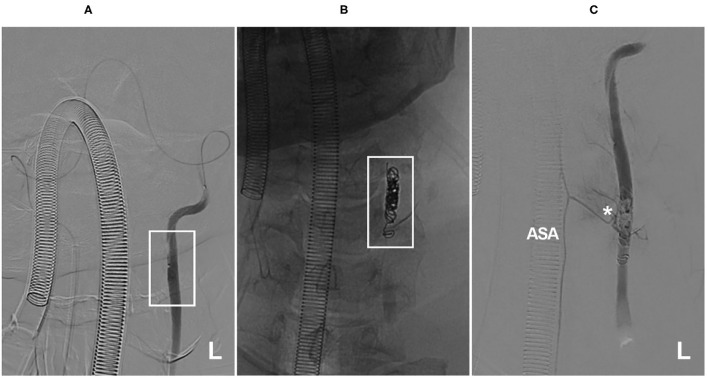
Insufficiency of the artery of cervical enlargement after VA occlusion. (A) A microcatheter angiogram showed that the microcatheter reached the left VA (frame) from the right to perform VA occlusion, and no segmental artery was observed. (B) X-ray film showed left VA occlusion by coiling (frame). (C) A microcatheter angiogram showed that the coils occluded the origin of the artery of cervical enlargement (asterisk). ASA, anterior spinal artery; L, left; VA, vertebral artery. The patient was a 56-year-old man. He suffered neck bleeding from the VA; thus, the VA was occluded. After the treatment, he had no new neurological deficits.
